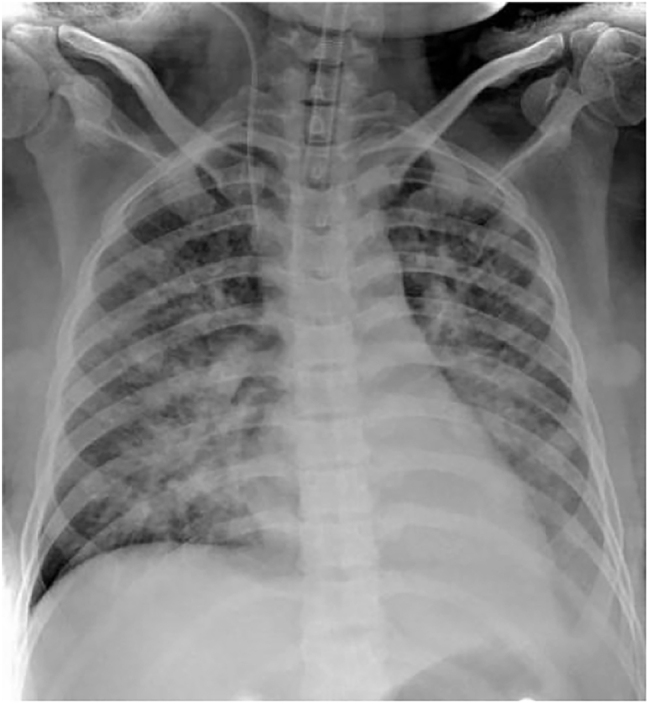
Chest X-ray chest X-ray. Bilateral lung field translucency is unevenly decreased, bilateral lung texture is increased and thickened, and multiple patches and speckled hyperdense shadows with unclear borders can be seen. Multiple foci of disease are present in both lungs, and the possibility of pulmonary edema is considered in conjunction with the clinical picture.
